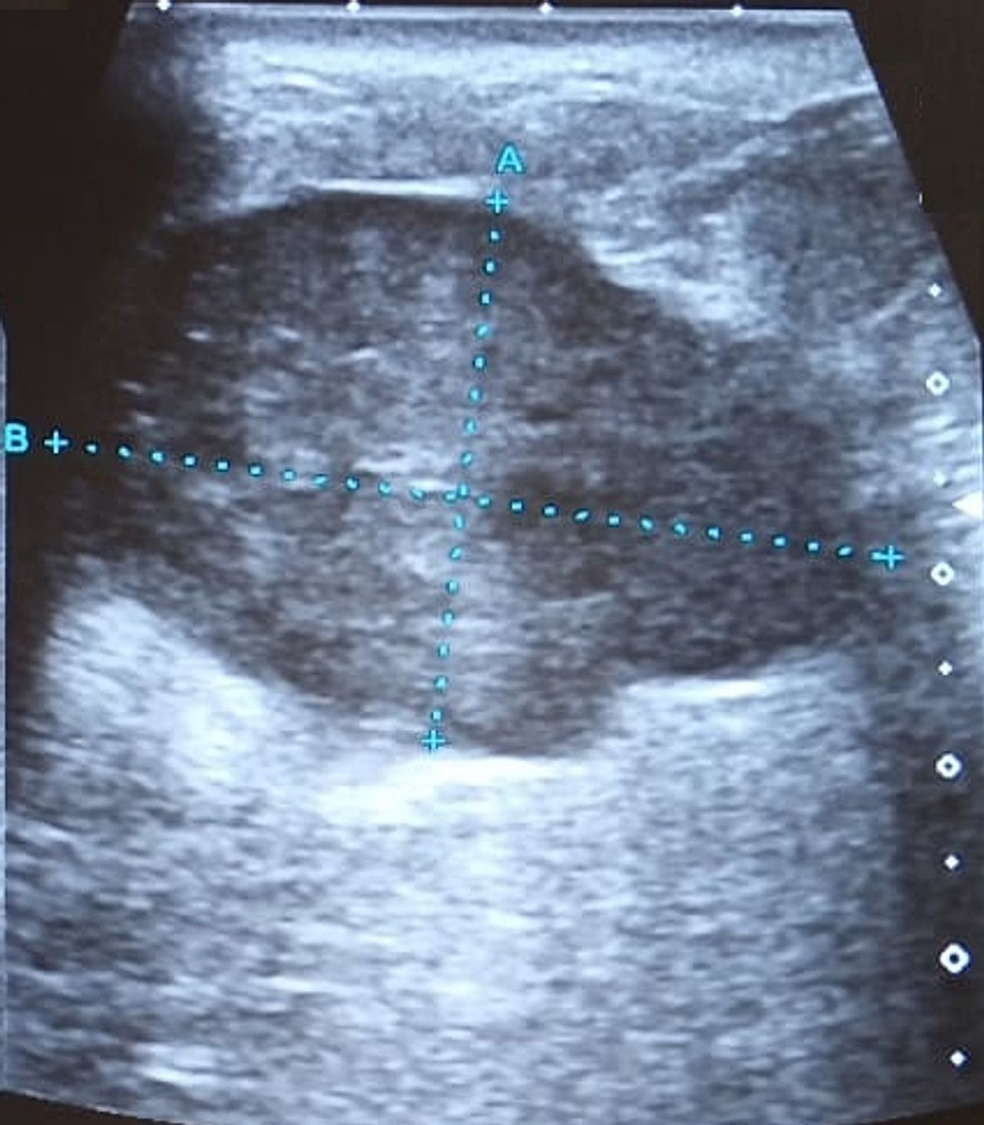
Ultrasonography of the breast indicated an ill-demarcated hypoechoic irregular lesion with thin fluid streaks as marked by the dotted lines A and B.
